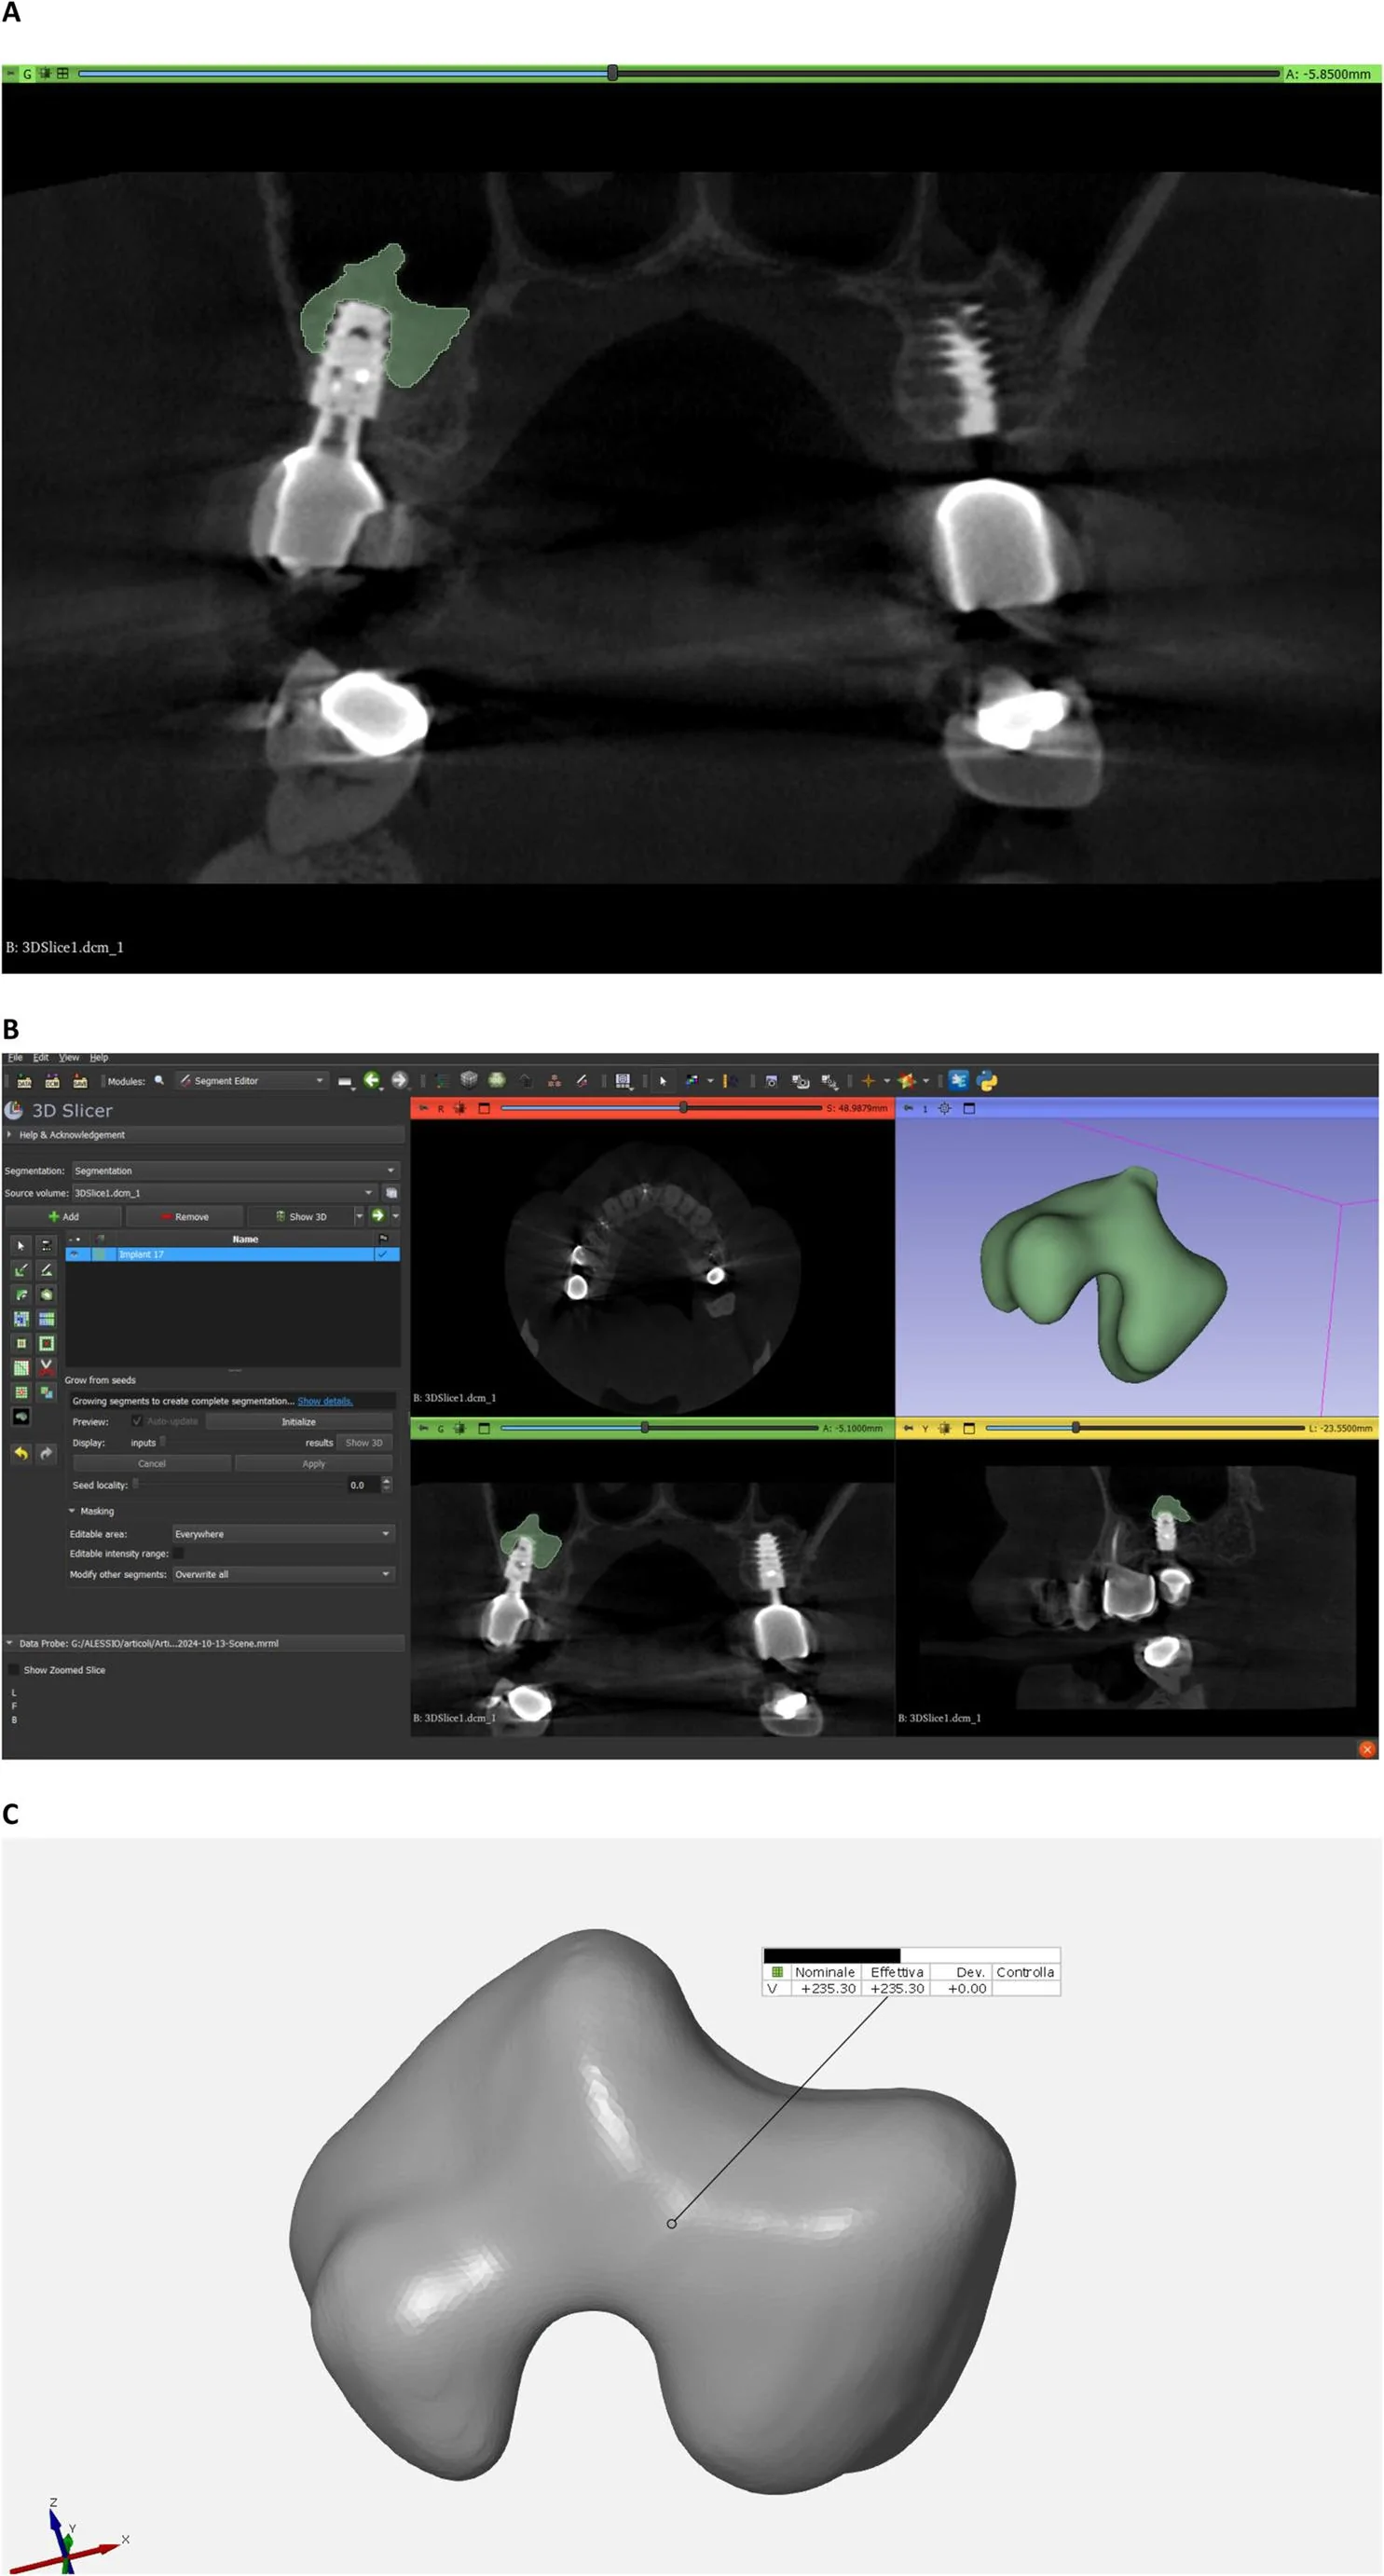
The post operative and pre operative CBCT scans were analyzed. The implant site was analyzed considering the ideal implant axis (as previously calculated through a 3D planning software – Real Guide, 3Diemme) (A). After the preliminary phase, the 1-year CBCT was analyzed with a specific segmentation software (3D Slicer, Harvard University). A semi-automatic segmentation technique was performed in order to create a stl file of the regenerated area (B) The volume of the regenerated area was calculated with a specific software (GOM Inspect, Zeiss) (C)
